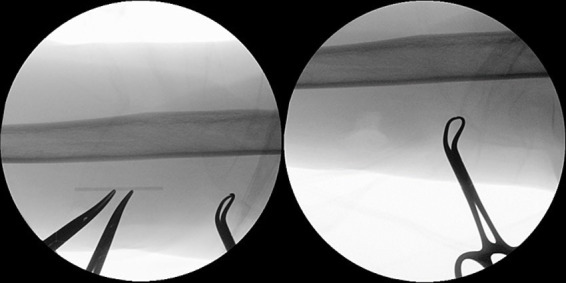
Successful retrieval of an intact 4 cm etonogestrel implant under fluoroscopic guidance after failed ultrasound-guided attempts in a 33-year-old woman (Busan, South Korea, 2025).
